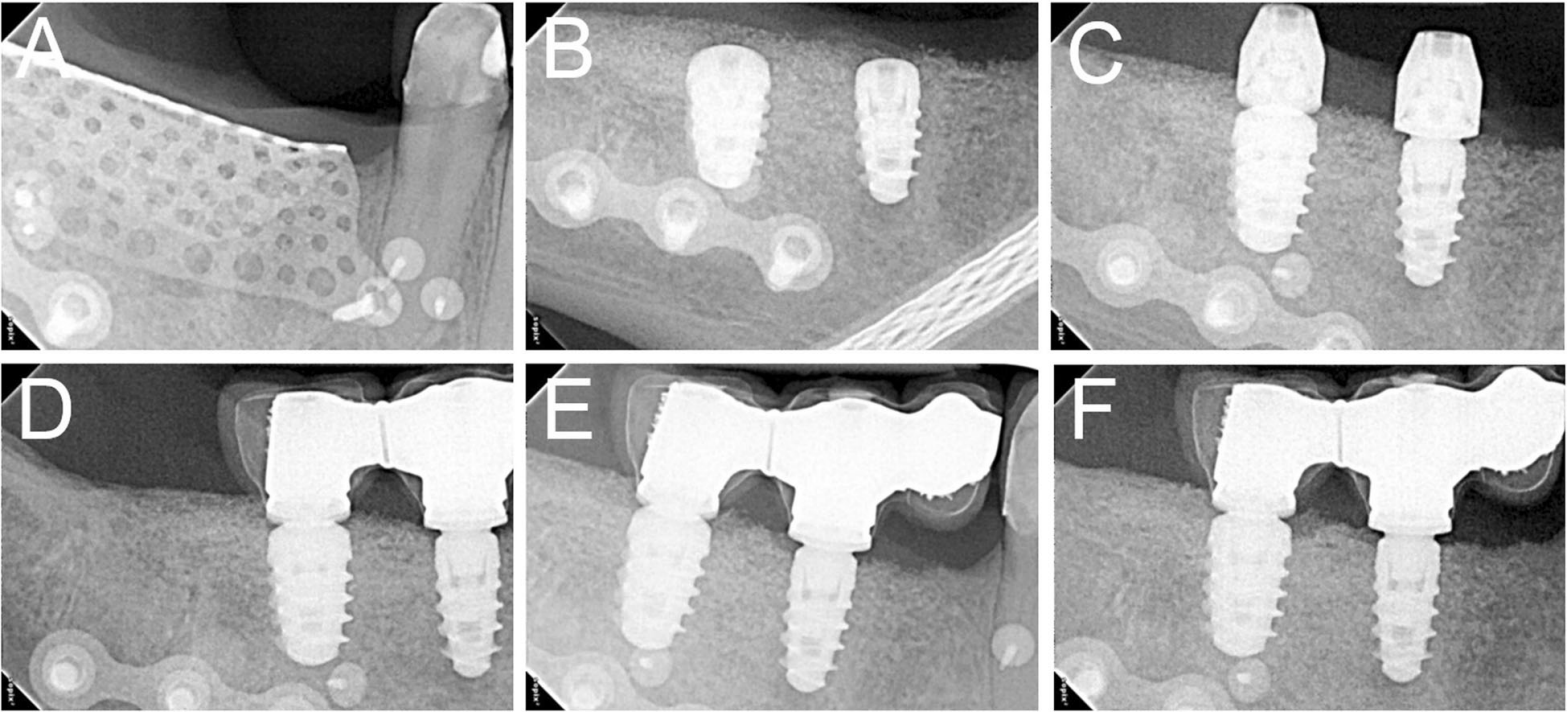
Radiographic images of the customized mesh in position (a), of the implants placed in regenerated bone (b), the abutments (c), and the delivery of the final restoration (d). Radiographic controls 6 months (e) and 1 year (f) after the delivery of final restoration
